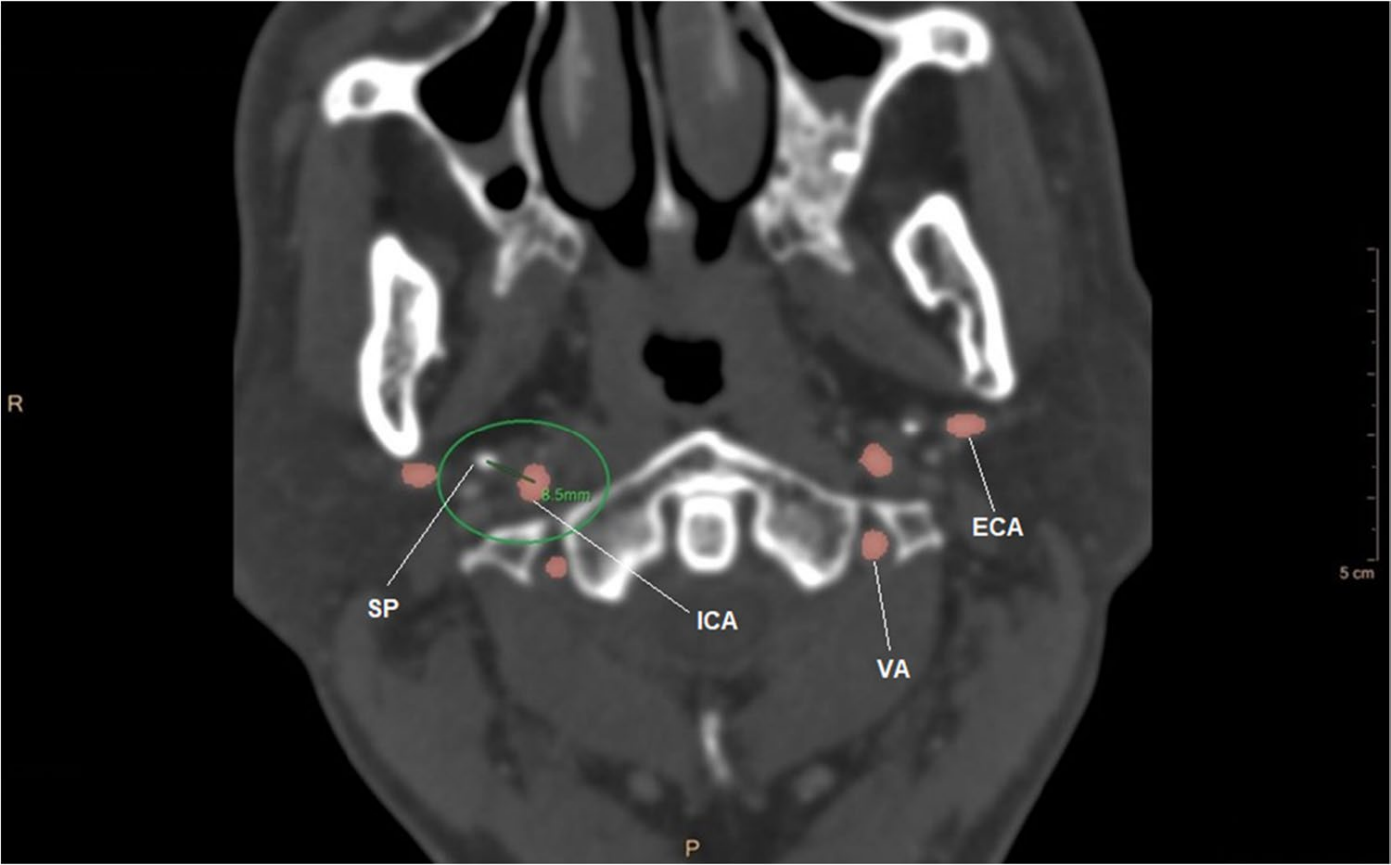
CT angiography image of intercentric distance (SP, styloid process; ICA, internal carotid artery; VA, vertebral artery; ECA, external carotid artery)the marginal distance, expressed in millimeters, from the edge of the vessel wall to that of SP (Fig. 4).
